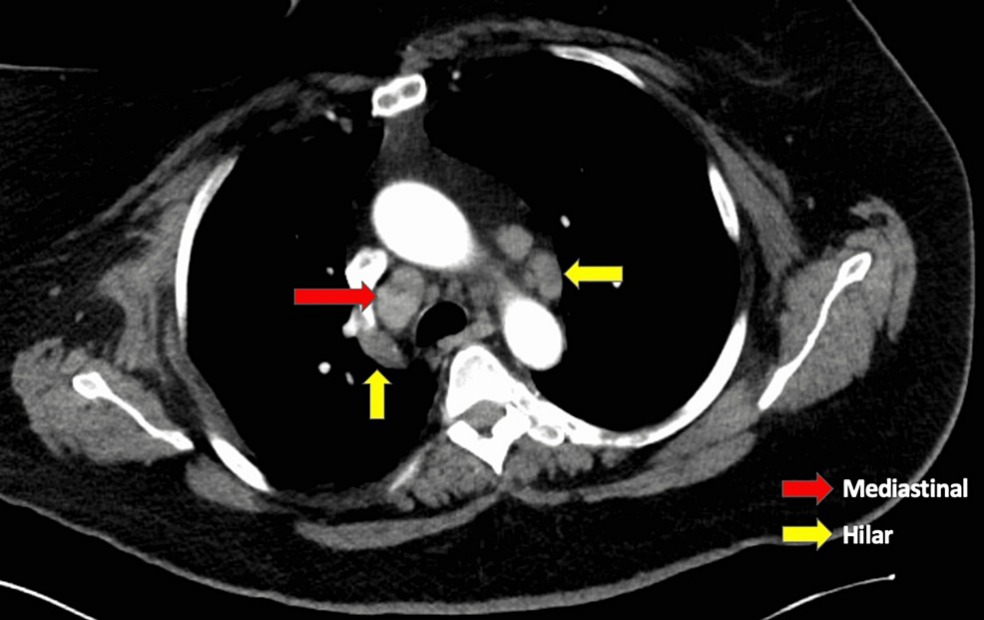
CT scan of the chest revealed mediastinal and hilar lymphadenopathy (red arrow indicating mediastinal nodes; yellow arrows indicating hilar nodes).
